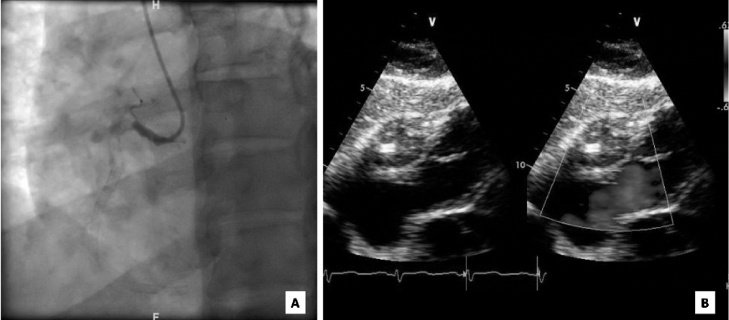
(a) Coronary angiogram showing complete right coronary artery occlusion. (b) Trans-thoracic echocardiogram with a tissue like mass seen in the lateral annulus of the tricuspid valve measuring 41 by 29 mm with an area of calcification in the center.
